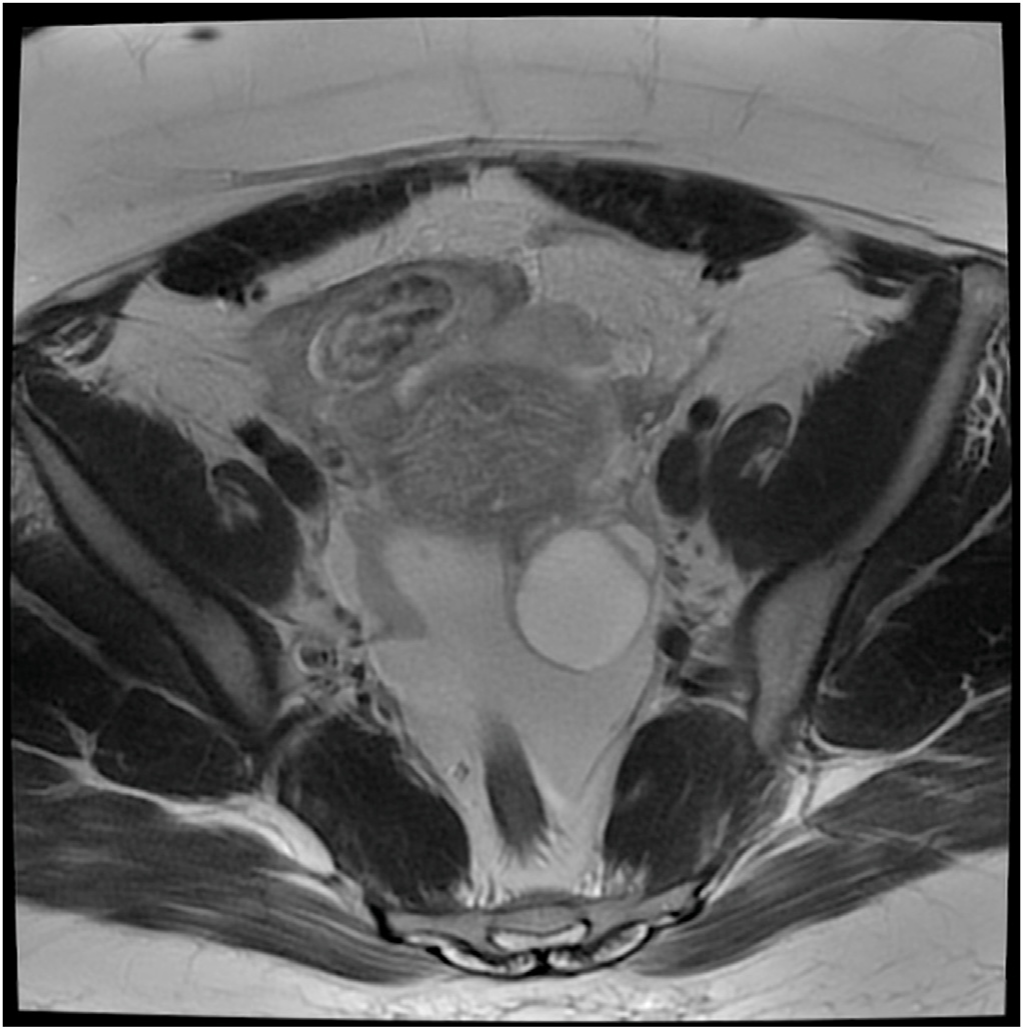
Axial T2 MRI demonstrates a right-sided tubal ectopic pregnancy with heterogenous internal signal. A low signal (white) cyst is noted from the left ovary. Reprinted from content created by Vikas Shah from Radiopaedia.org, rID:87383, under CC BY-NC-SA 3.0 license (https://creativecommons.org/licenses/by-nc-sa/3.0). MRI, magnetic resonance imaging.
